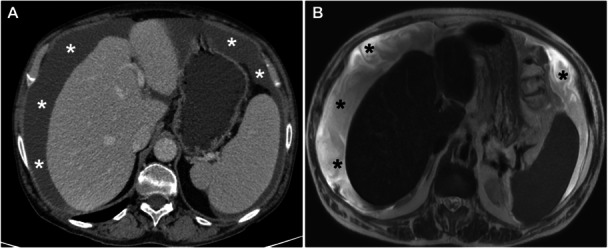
Female, 82‐year old, on computed tomography portal venous phase (A) and magnetic resonance imaging T2w (B), affected by colon cancer with peritoneal carcinomatosis and ascites (white and black stars in A and B, respectively).
